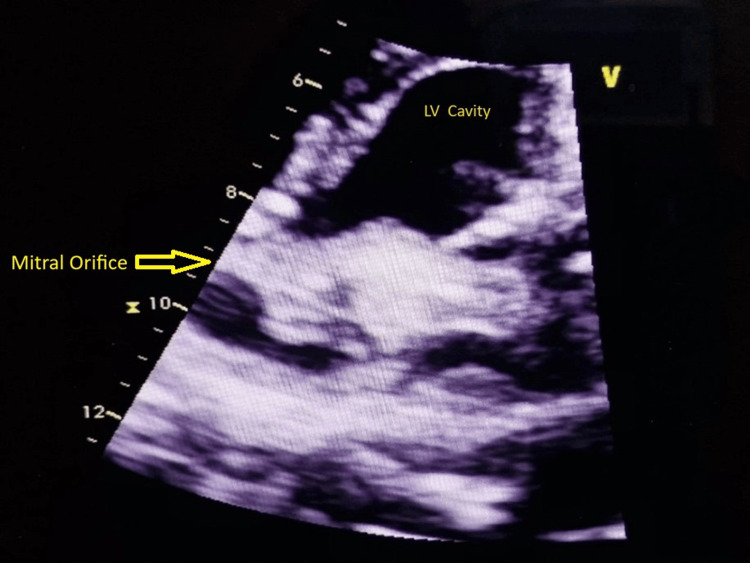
Echocardiography showing obstructive thrombus on mechanical mitral valve in PSAX at mitral level during first presentation PSAX - parasternal short axis view; LV - left ventricle
